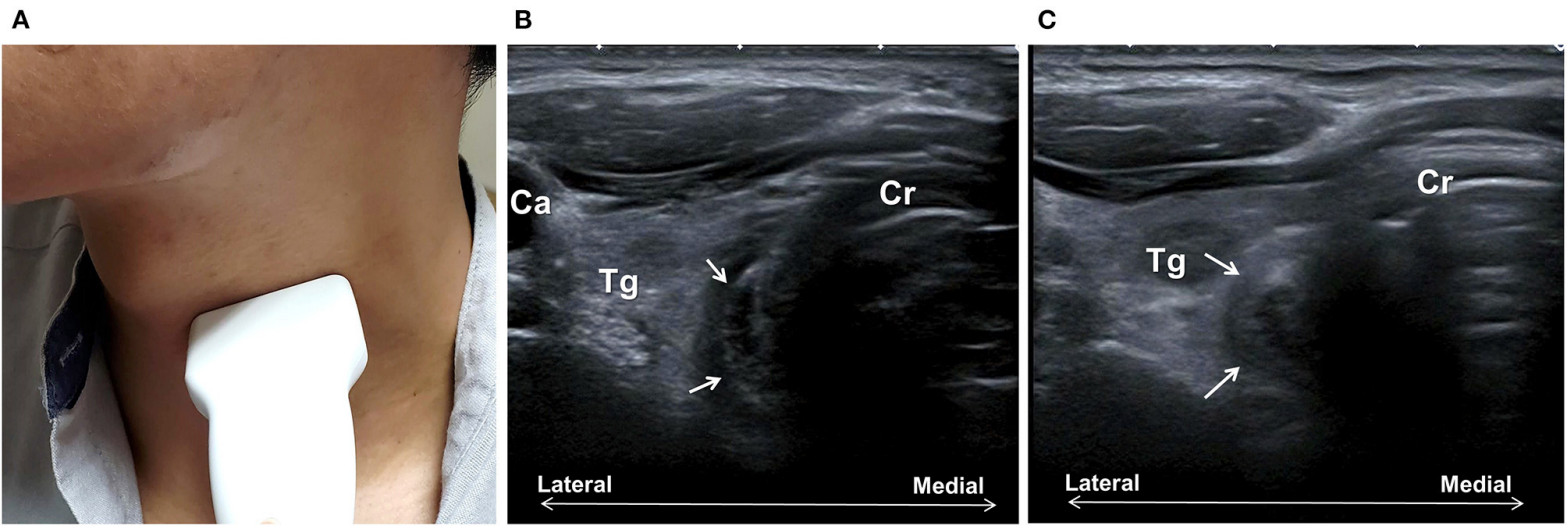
Ultrasonography assessment of the cricopharyngeal muscle (CPm). The transducer is placed transversely at the left of the trachea at the cricoid cartilage (Cr) level (A). The CPm (arrows) appears as an oval-shaped multiplayer tubular structure (B). Bolus passing can be observed with the rounding of the CPm during swallowing (C). Ca, common carotid artery; Tg, thyroid gland.
